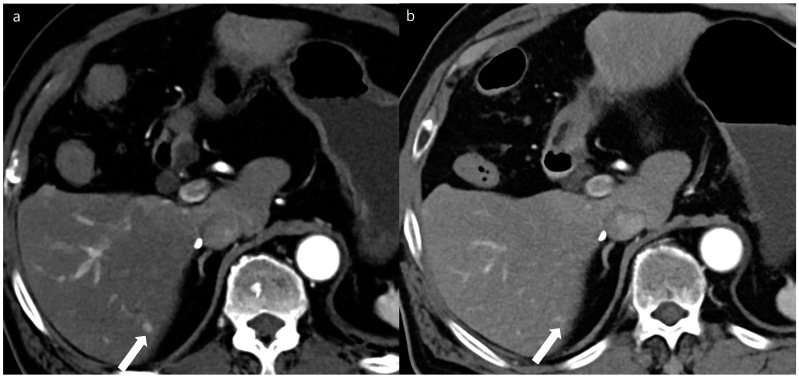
An 82-year-old man with a 0.5 cm sized enhancing nodule at segment 6 of the liver (arrows). A double low-dose CT using a deep-learning-based contrast-boosting model (a) shows better conspicuity (Reviewer 1, 5; Reviewer 2, 4) of the focal lesion (arrows) than standard-dose CT using hybrid iterative reconstruction (Reviewer 1, 5; Reviewer 2, 3) (b) at arterial phase images (time interval: 4 months). Two reviewers evaluated the double low-dose CT using deep-learning-based contrast-boosting model and standard-dose CT using hybrid iterative reconstruction. The results demonstrated no difference in overall image quality.
